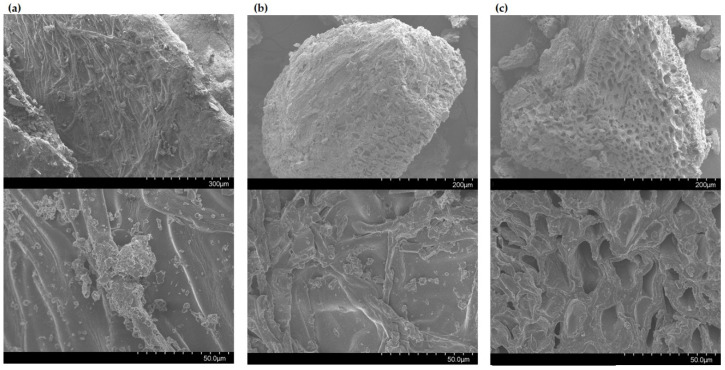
SEM images of *F. vesiculosus* (a) untreated samples (intact and dried macroalgae), (b) control samples (50% ethanol, 30 min, no ultrasound) and (c) UAE treated samples (35 kHz, 50% ethanol, 30 min). Scale bars: 300 µm (magnification 150×), 200 µm (magnification 250×) and 50 µm (magnification: 1000×).
